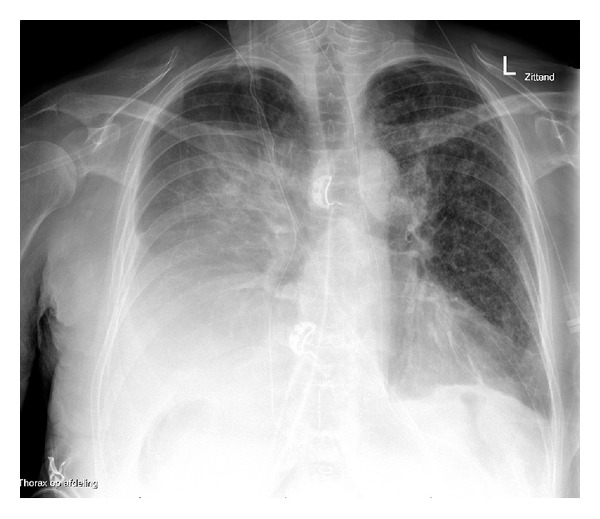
Chest radiography showing unilateral pleural effusion.
